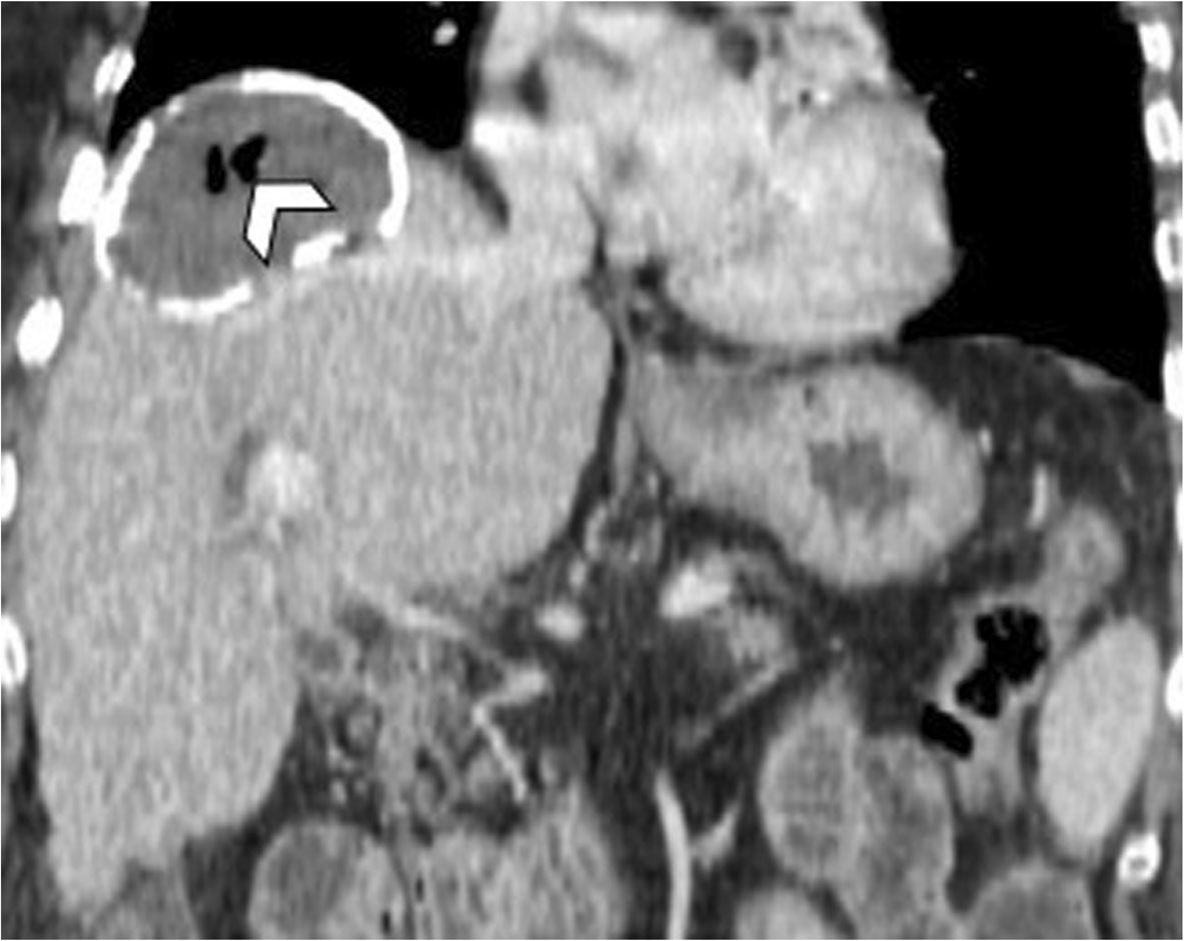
A 54-year-old man with air content within a hepatic hydatid cyst. Coronal reformatted CT image on portal venous phase shows air bubbles (arrowhead) within a unilocular calcified hydatid cyst. This finding was suggestive of occult cysto-biliary communication, which was later confirmed at surgery
